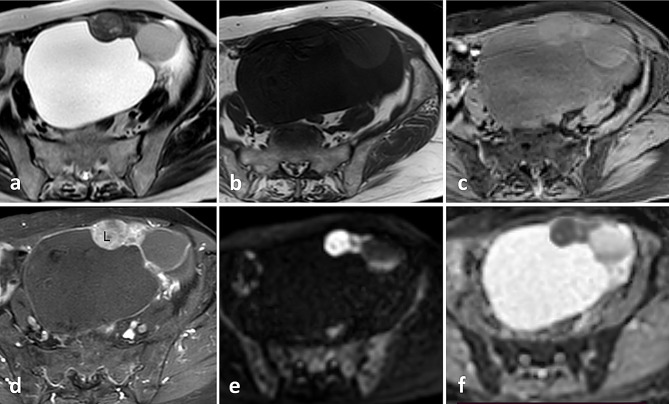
A left ovarian O-RADS MRI score 5 lesion. (a) Axial T2-WI reveals midline multilocular cystic adnexal mass with a large solid tissue of intermediate signal intensity. (b) Axial T1-WI revealed intermediate signal intensity locule without a signal drop on T1 fat-saturated image (c), denoting hemorrhagic content (d) Axial T1 contrast-enhanced images reveal avidly enhancing solid lesion (L) more than the myometrium (M) at 30–40 s. (e) Axial DWI at b = 1000 reveals a high signal of the solid tissue and hypointensity at the corresponding ADC image, denoting a restricted diffusion pattern. Pathology revealed ovarian high-grade serous carcinoma
